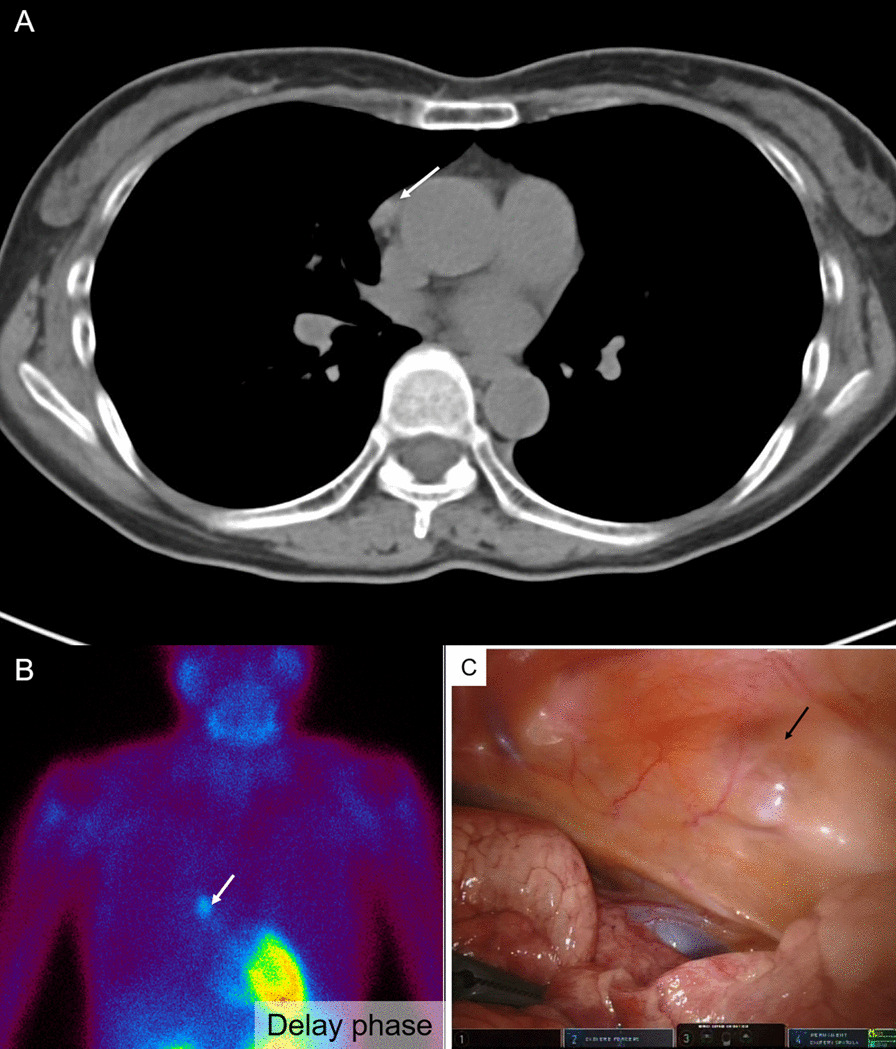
Computed tomography (CT) imaging and Technetium-99m-methoxy-isobutyl-isonitrile (99mTc-MIBI) scintigraphy. A Chest CT showed anterior mediastinal nodule (white arrow). B 99mTc-MIBI scintigraphy showed no accumulation at delay phase in the thyroid gland, but accumulation in the anterior mediastinal nodule (white arrow). C Intraoperative findings. The location of the tumor (black arrow) was immediately identifiable
